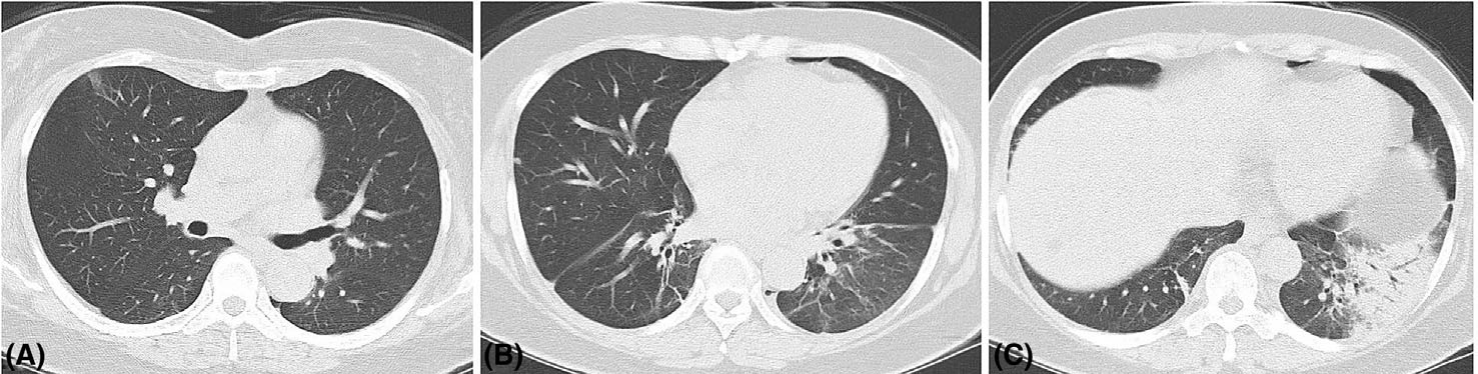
CT findings of a typical case (Case 7, female, 59 years old). (A) Small patchy ground‐glass density shadows in the subpleural right lung upper lobe. (B) Small patchy consolidation shadows in the subpleural right lung middle lobe. (C) Consolidations, ground‐glass density shadows, and air bronchogram in the left lung inferior lobe, and consolidation shadows in the right lung inferior lobe.
